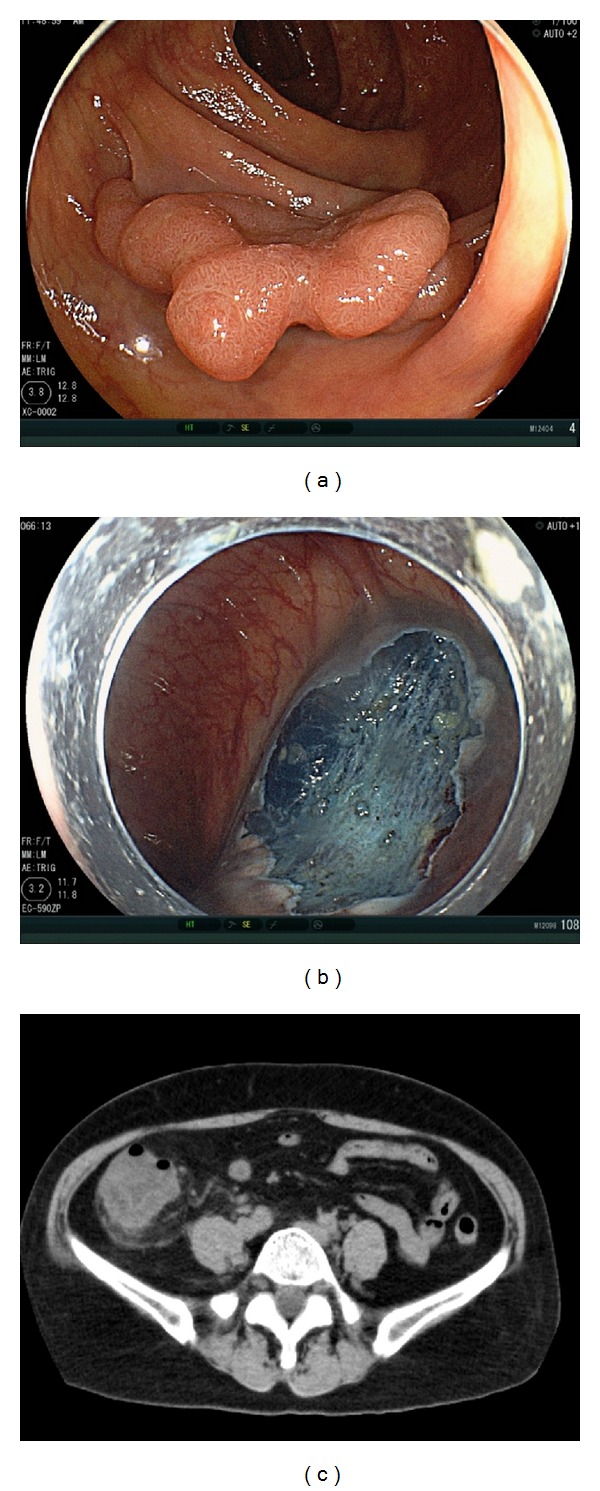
(a) Colonic tumor, 0-IIa, 40 mm in diameter, located in the ascending colon. (b) The ulceration after endoscopic submucosal dissection (ESD). ESD was performed without perforation and coagulation of the muscle layer. (c) Abdominal computed tomography 2 days after ESD revealed wall thickness on the whole ascending colon.
